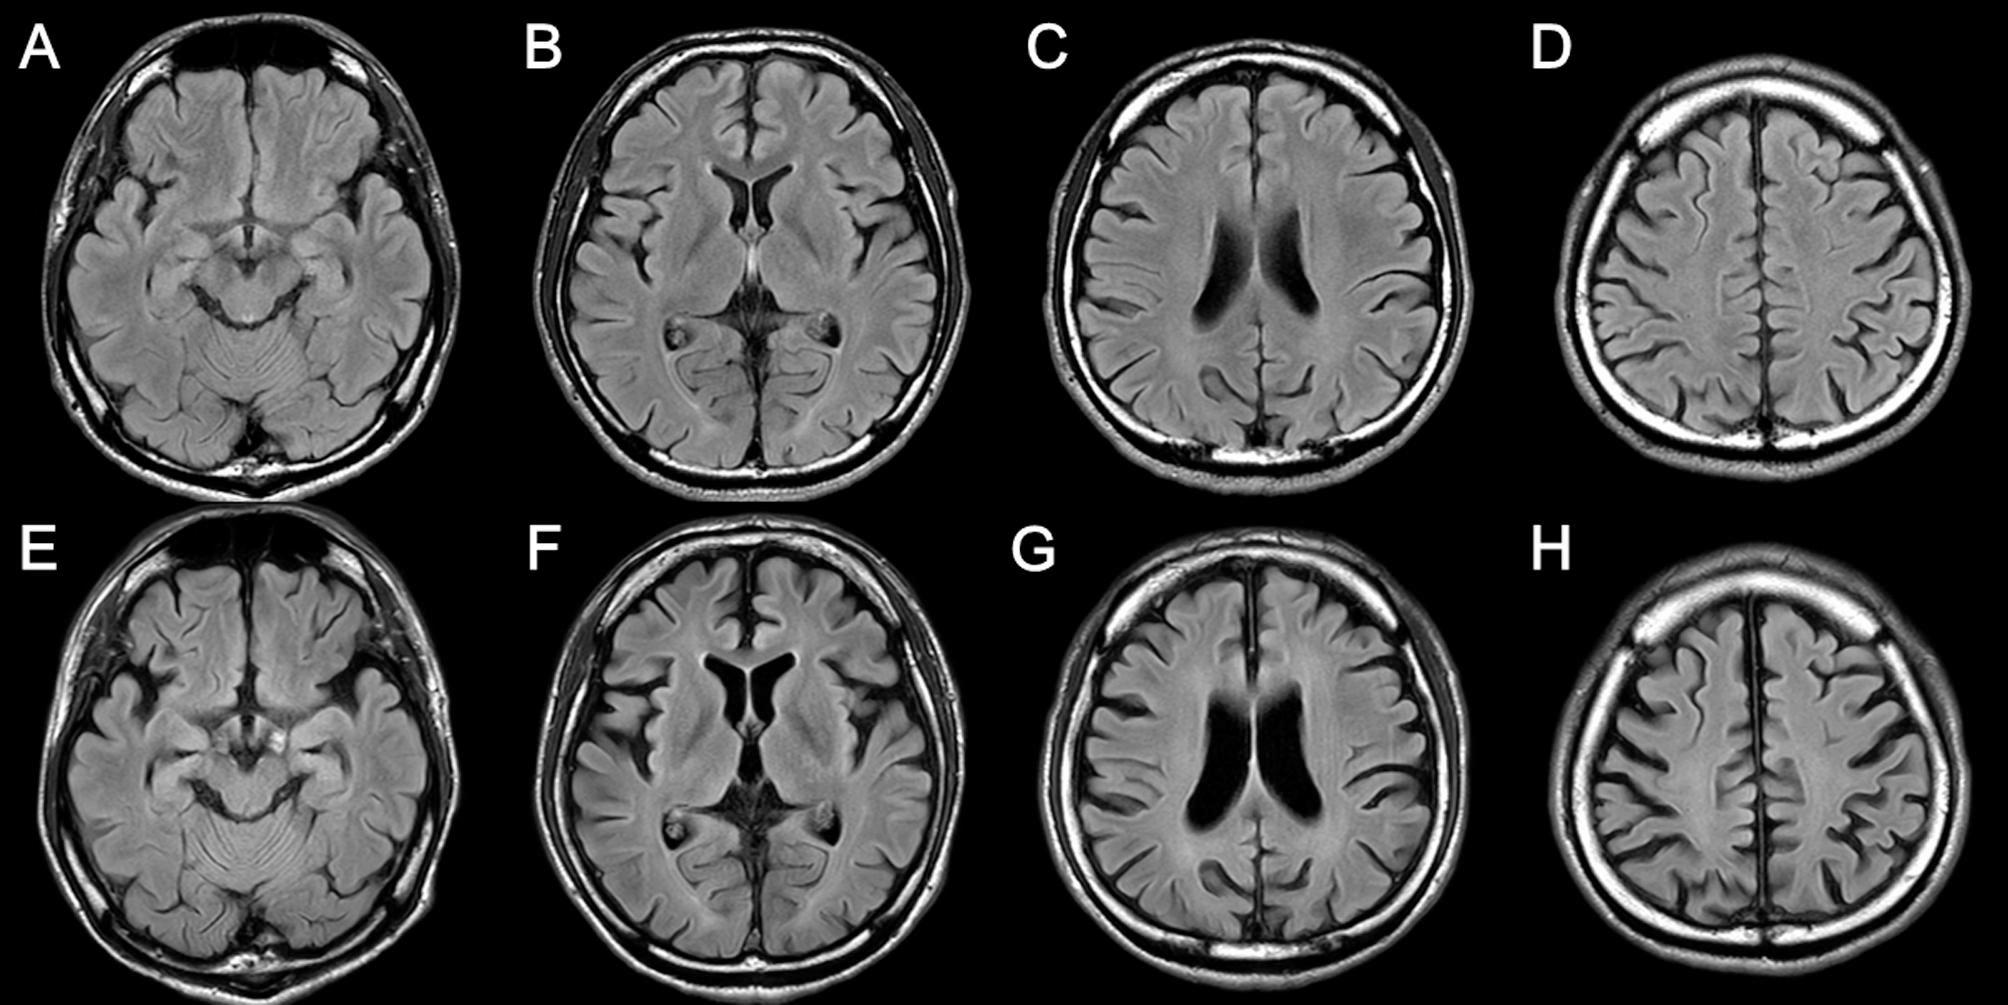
Brain magnetic resonance imaging (MRI) findings. Fluid-attenuated inversion recovery (FLAIR) images of brain MRI obtained at the first visit (23 years old) (A–D) showed mild diffuse brain atrophy. The FLAIR images of follow-up brain MRI obtained at 4 years after the initial one (E–H) showed more advanced diffuse brain atrophy
